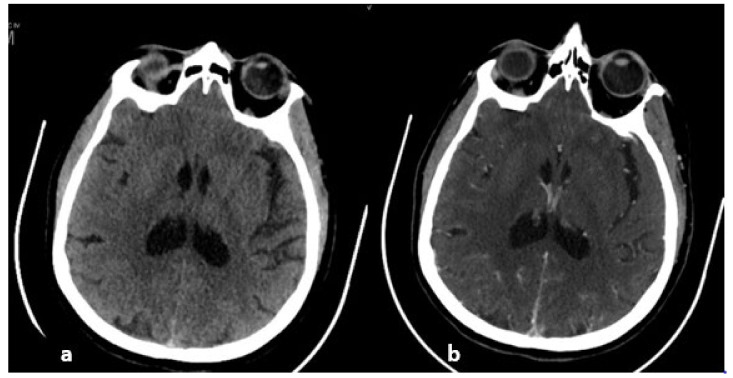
CT. From left to right: (a) nonenhanced CT: spontaneous hyperdensity of the superior sagittal sinus, (b) enhanced CT: empty delta sign testifying to the circulation of contrast around the thrombus, which appears as a defect of signal in the center of the superior sagittal sinus.
